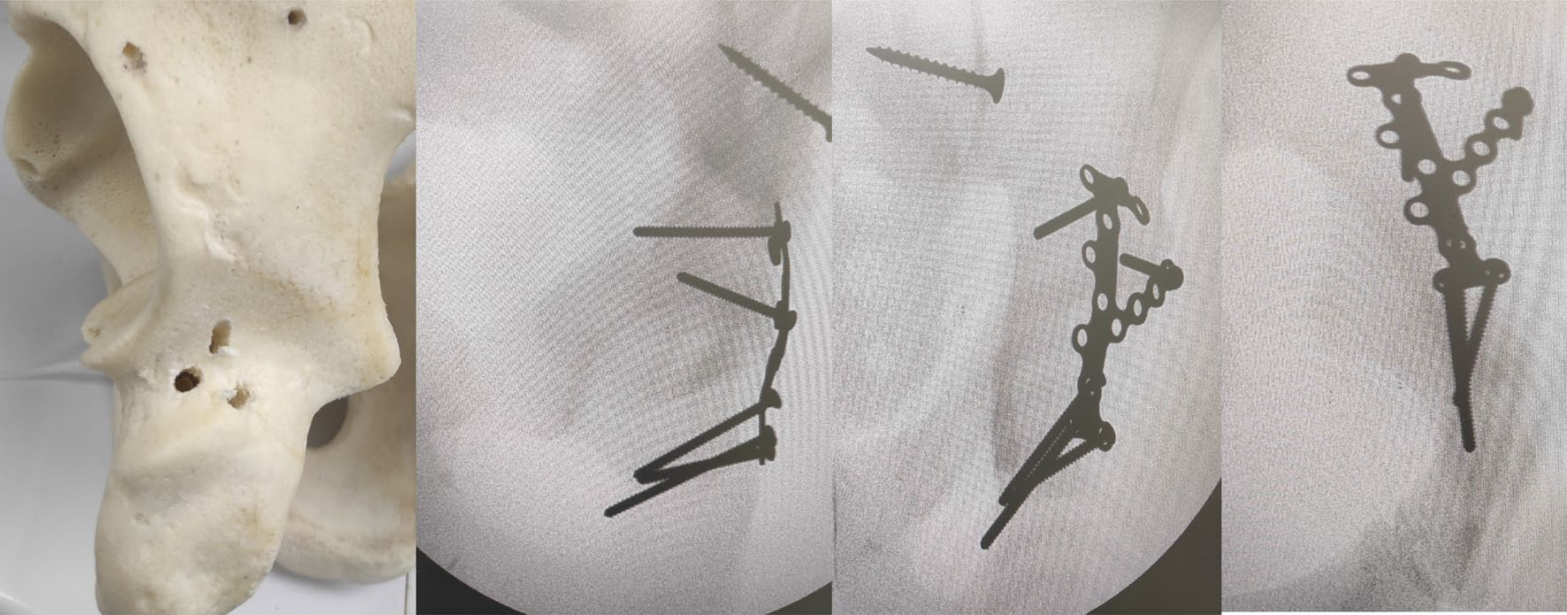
Saw bone and its radiograph demonstration showing 3 ischial screws can be inserted in a triangular pattern at the ischial tuberosity upper end together with the V shape anchorage of the proximal part of the plate
